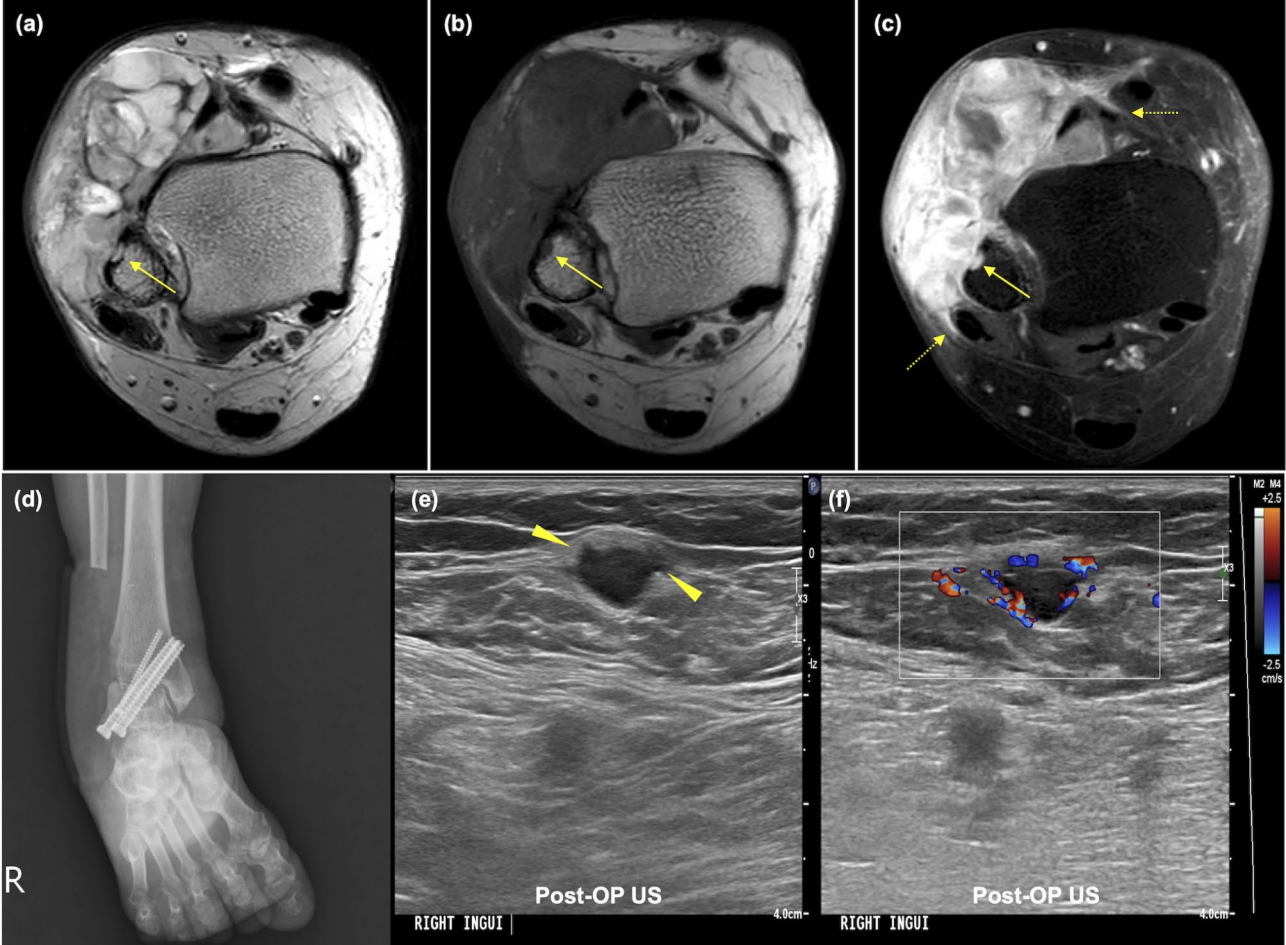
Detection of metastatic lymphadenopathy through short-term USG in a 69-year-old female with undifferentiated pleomorphic sarcoma. (a,b,c) Axial T2-, T1-, and enhanced T1-weighted images revealed infiltrative heterogeneous signal intensity mass with enhancement primarily located in the subcutaneous layer of the right anterolateral ankle. Focal invasion with cortical erosion at the distal fibula was noted (arrows). Additionally, the mass exhibited tail-like enhancement along the investing fascia (dashed arrows). (d) The patient underwent wide excision of the tumor with fibular resection and tibiotalar fusion. (e) On her postoperative USG, 21 months after wide resection, a small but suspicious-looking lymph node was incidentally observed in the right inguinal area. Loss of hilar echogenicity and infiltrative growth of the tumor outside the original lymph node contour were observed (arrowheads). (f) Color Doppler USG revealed peripheral vascularity. The lymph node was excised and confirmed as a metastatic lymph node
